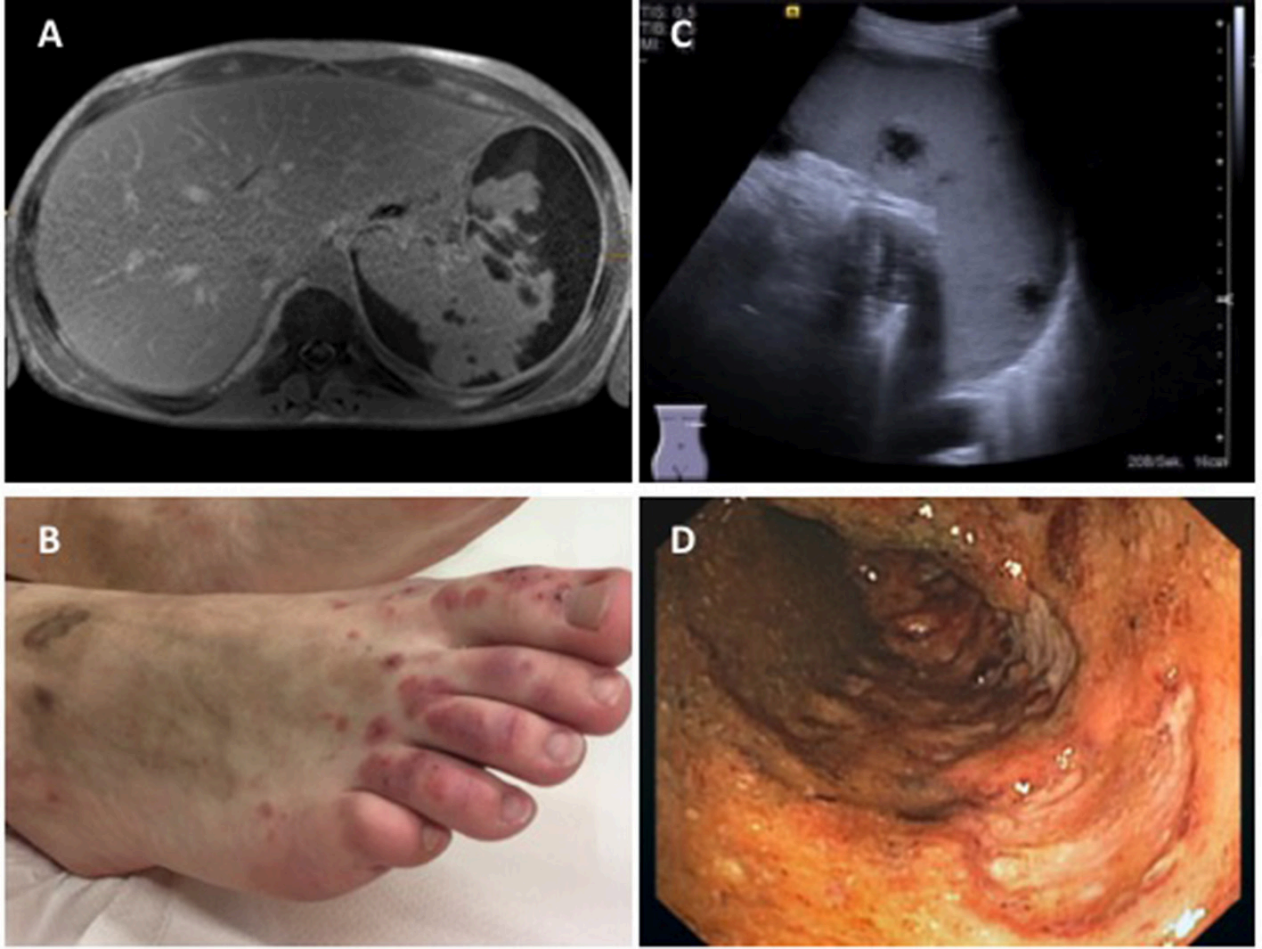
Findings in a 16-year-old boy with GPA. (A) Abdominal MRI showing pronounced parenchymatous necrosis of the spleen with distension of the spleen capsule (provided by Dr. Gabriele Hahn, Pädiatrische Radiologie, Universitätsklinikum Carl Gustav Carus, TU Dresden); (B) Necrotizing skin vasculitis on legs and feet; (C) Splenomegaly, spleen with increased echogenicity, subcapsular fluid accumulation, hypoechogenic necrotic areas with absence of perfusion (provided by Dr. Heike Taut, Klinik und Poliklinik für Kinder- und Jugendmedizin, Universitätsklinikum Carl Gustav Carus, TU Dresden); (D) Severe enterocolitis (shown: transversal colon) with deep ulcerations as a result of necrotizing vasculitis (provided by Dr. Martin Laass, Klinik und Poliklinik für Kinder- und Jugendmedizin, Universitätsklinikum Carl Gustav Carus, TU Dresden). Consent of the patient was obtained to publish these images.
